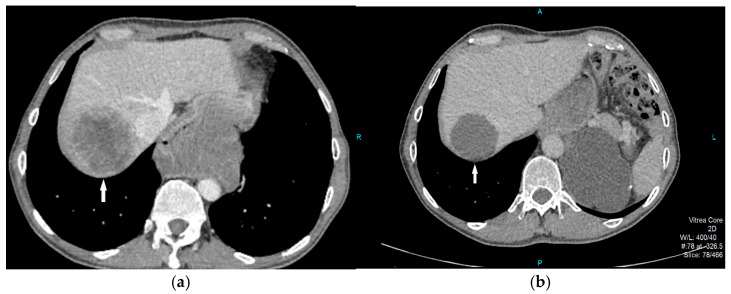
CT axial scans show the preoperative appearance of the metastasis (a) in which the solid component of the tumor with a central zone of necrosis dominates (white arrow), while the second image two years later (b) clearly shows the positive effect of the implemented therapy, with a predominantly cystically degenerated lesion (white arrow) with dimensional regression of up to 1 cm. R—right, A—anterior, L—left.
